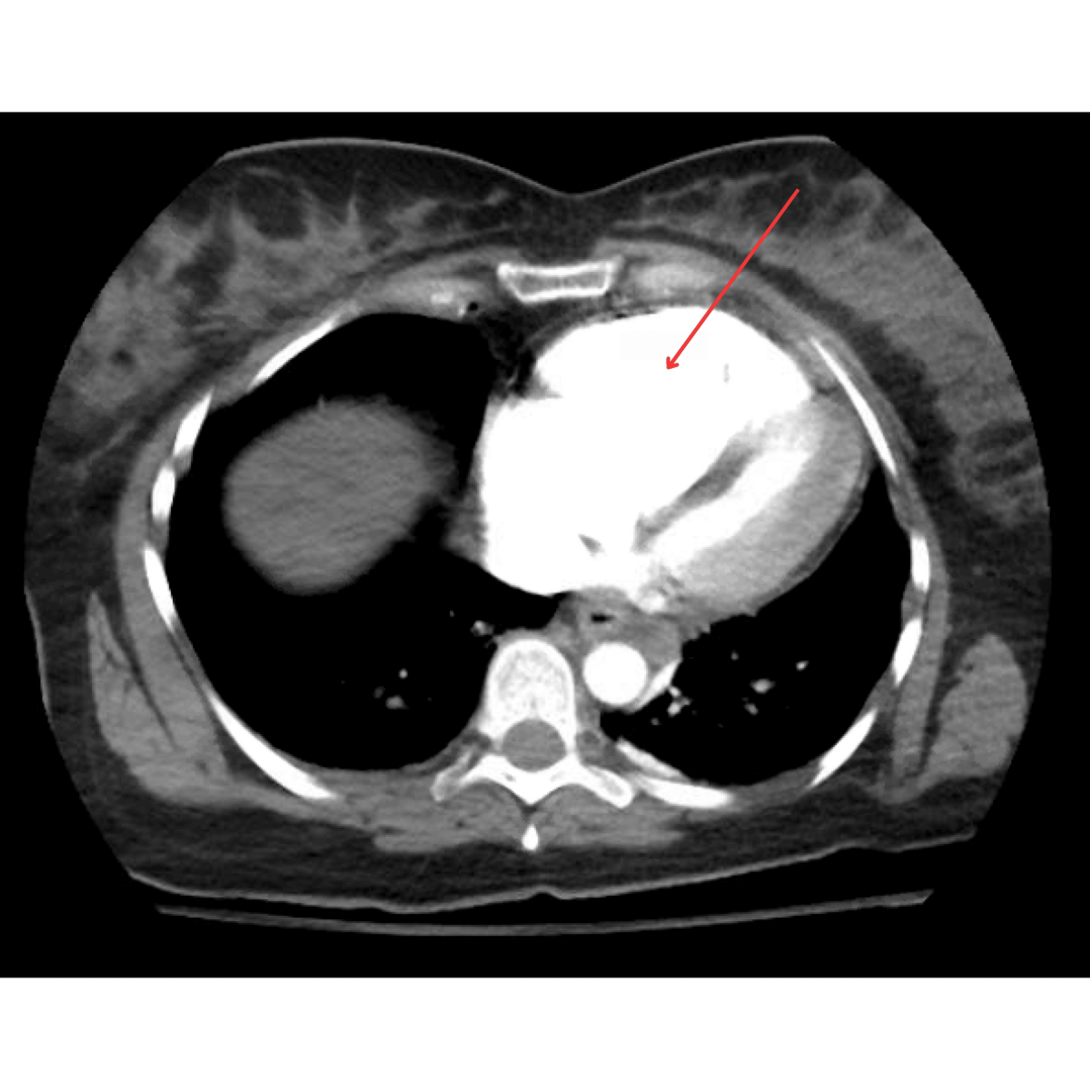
CTA chest pulmonary embolism CTA: computed tomography angiography The right ventricle to left ventricle ratio was measured as greater than 1.0, with findings suggestive of right heart strain in the setting of identified pulmonary emboli.
